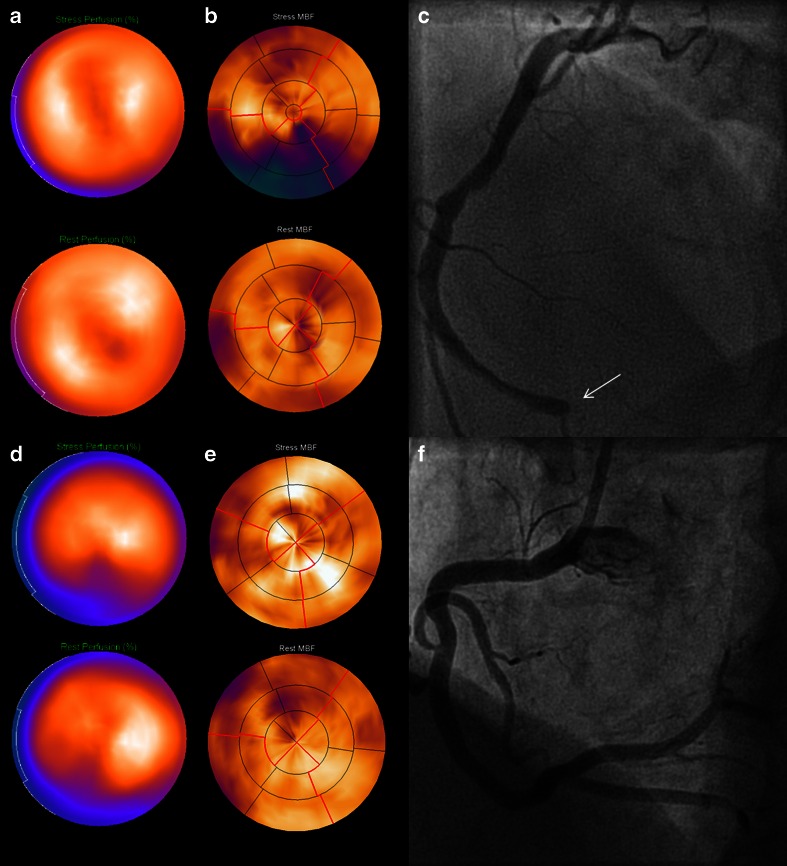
Representative cases of a false-negative and false-positive SPECT result due to limited tracer extraction or attenuation, respectively. False-negative SPECT result (a) in case of an unmistakable perfusion defect of the inferior wall on PET (b) of a patient with a chronic total occlusion (*white arrow*) of the RCA on ICA (c). False-positive SPECT result (d) (perfusion defect of the inferior wall) of a patient with normal perfusion on PET (e) and no obstructive coronary artery disease on ICA (f). *SPECT* single photon emission computed tomography, *RCA* right coronary artery, *ICA* invasive coronary angiography; other abbreviations as in Fig. 2
